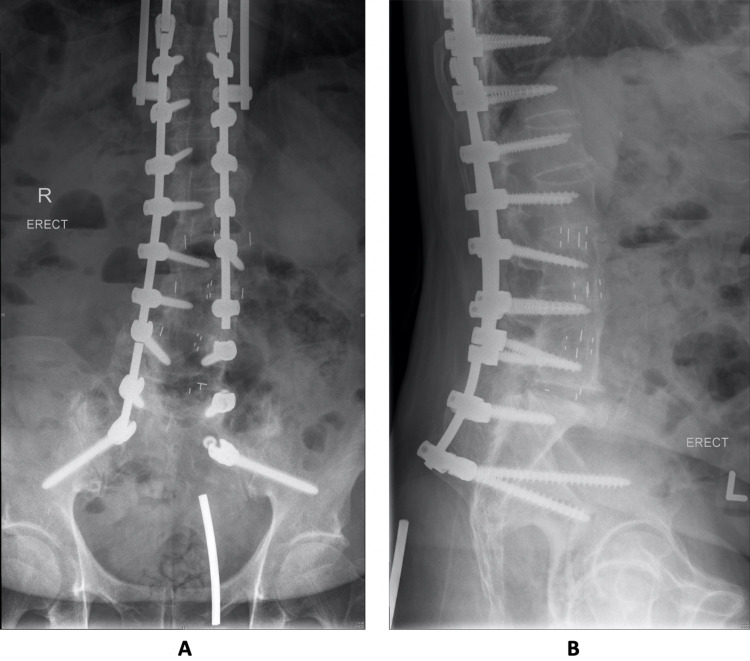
A: AP radiograph of the lumbar spine demonstrating broken rods bilaterally with migration of the rod on the left. B: Lateral radiograph of the lumbar spine demonstrating caudal migration of the rod into the soft tissue. AP, anterior-posterior
